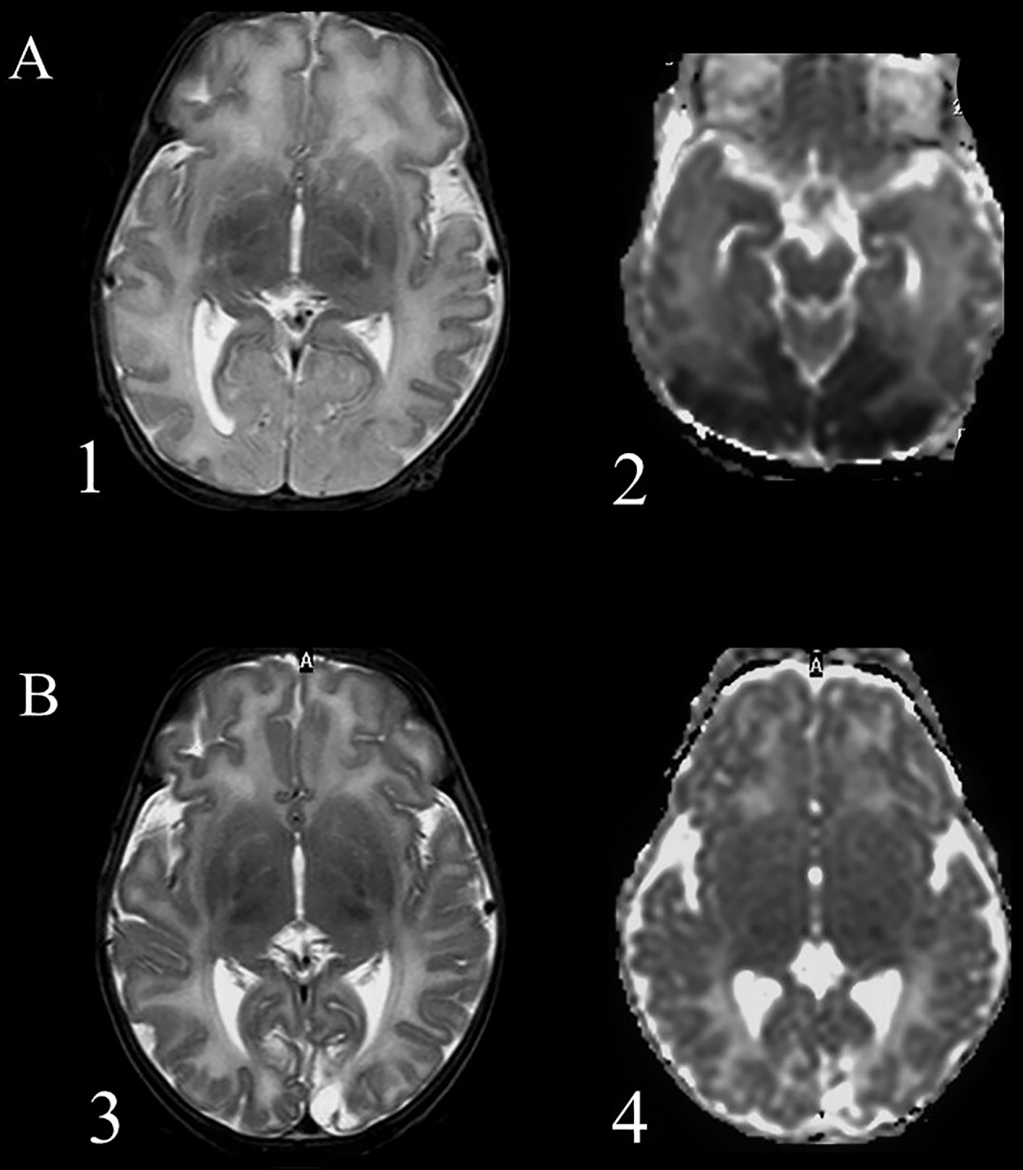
Magnetic resonance imaging of a term newborn with apnea and seizures associated with severe hypoglycemia. (A) scans performed after 5 days from the event (acute phase), 1 = axial T2 weighted imaging showing an extensive cortical and subcortical damage, manly in the occipital lobes, with loss of cortical differentiation; 2 = Diffusion weighted imaging shows a large posterior subcortical area of reduction of the apparent diffusion coefficient. (B) scans performed after 5 weeks (chronic phase): 3 = Presence of an occipital atrophic area, more extended within the left occipital lobe; 4 = Diffusion weighted imaging shows an increased water diffusion in the affected areas and a smaller cavitation.
